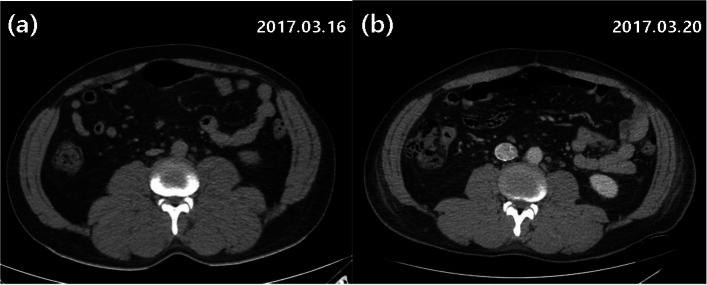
a Abdomen and pelvis CT demonstrates generalized edema of abdominal wall muscles. b Decreased muscle edema on subsequent chest CT 4 days later
